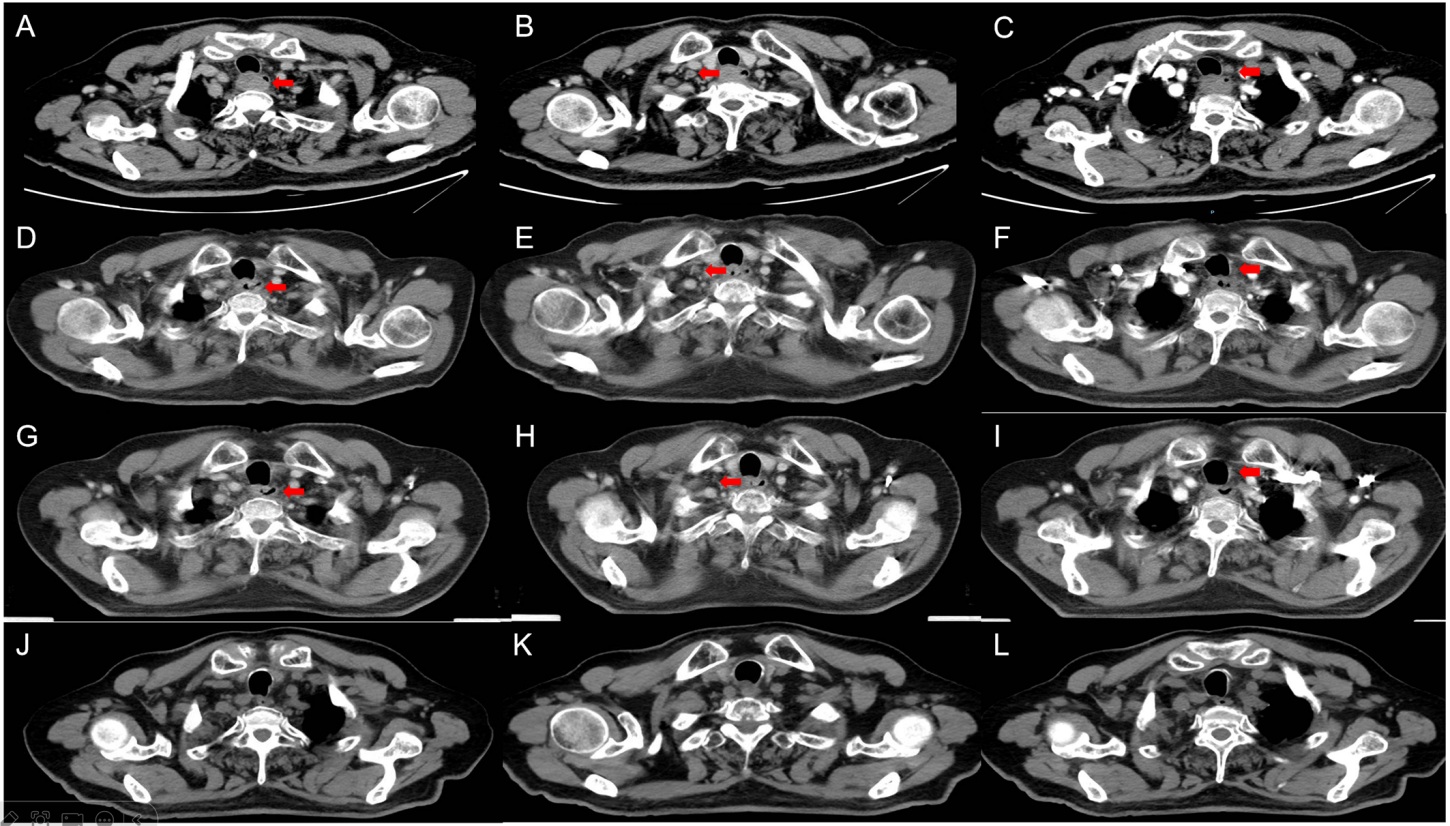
CT images during treatment. (A–C) CT images of the lesions at the initial treatment: esophageal lesions (A), supraclavicular lymph node lesion (B), and paratracheal lymph node lesion (C). (D–F) CT images after nine treatment sessions. (G–I) CT images at the end of radiotherapy. (J–L) CT images from the follow-up examination on July 30, 2024.
